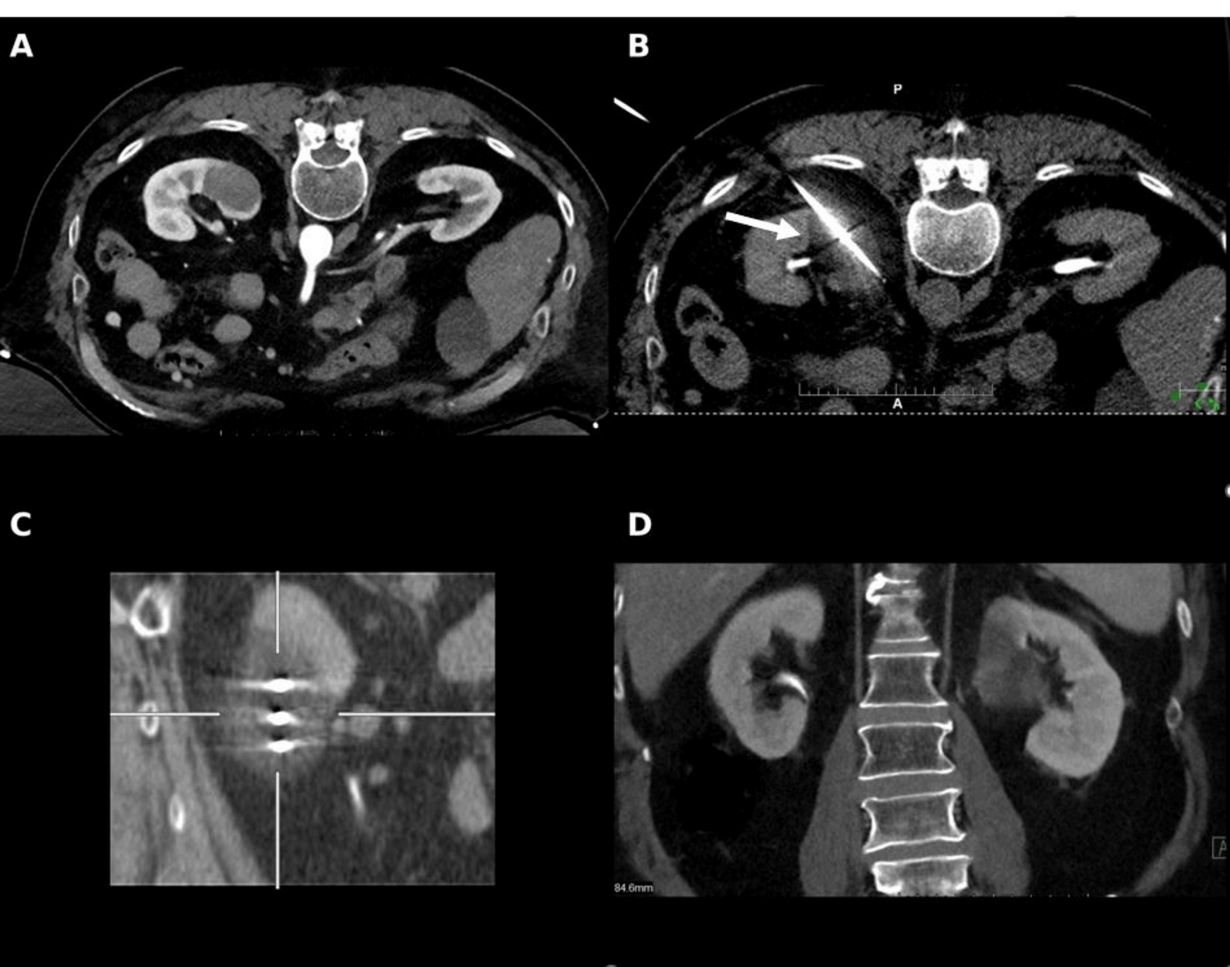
CT-guided percutaneous cryoablation of the left renal metastasis (A) Pre-ablation contrast-enhanced axial CT image used for trajectory planning and localization of the left renal lesion; (B) Intraprocedural axial CT showing cryoprobe placement and developing ice-ball (white arrow) encompassing the lesion; (C) Sagittal intraprocedural CT demonstrating all three cryoprobes within the tumor and the extent of ice-ball formation; (D) Coronal post-ablation CT demonstrating complete coverage of the lesion, consistent with successful ablation.
